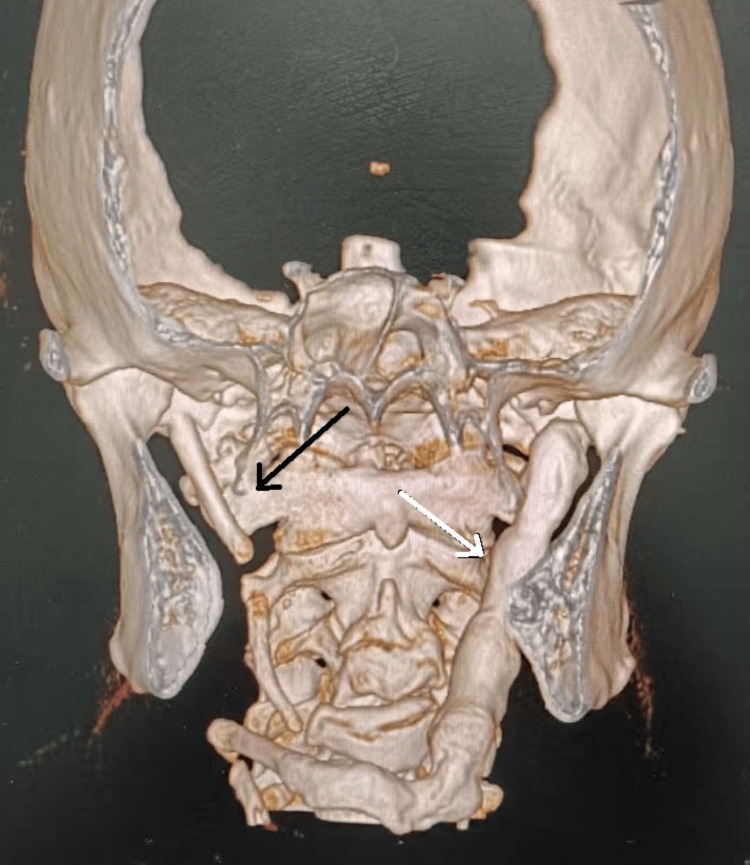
3D Cone-beam computed tomography (CBCT) reconstruction showing bilateral elongation of the styloid processes, right (black arrow) and left side (white arrow).
